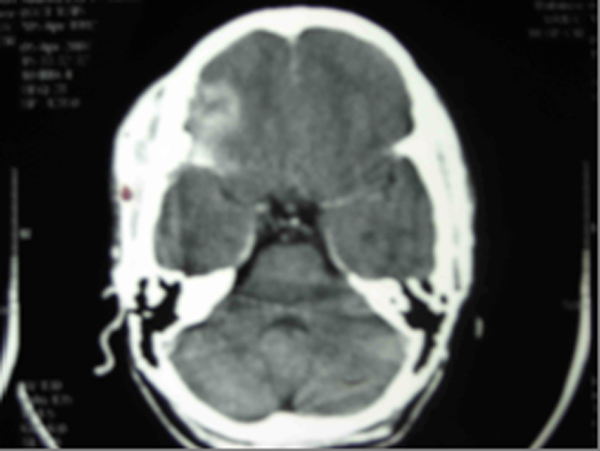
A computed tomography scan of the head showing a diffuse, homogeneously ill defined hyperdense lesion of size 2.9 × 5.3 cm (anteroposterior × thickness) involving the right orbit in its superior aspect with inferior and downward displacement of the right globe with extension into the anterior cranial fossa involving the right frontal region and the basal cistern region mainly on the right side.
